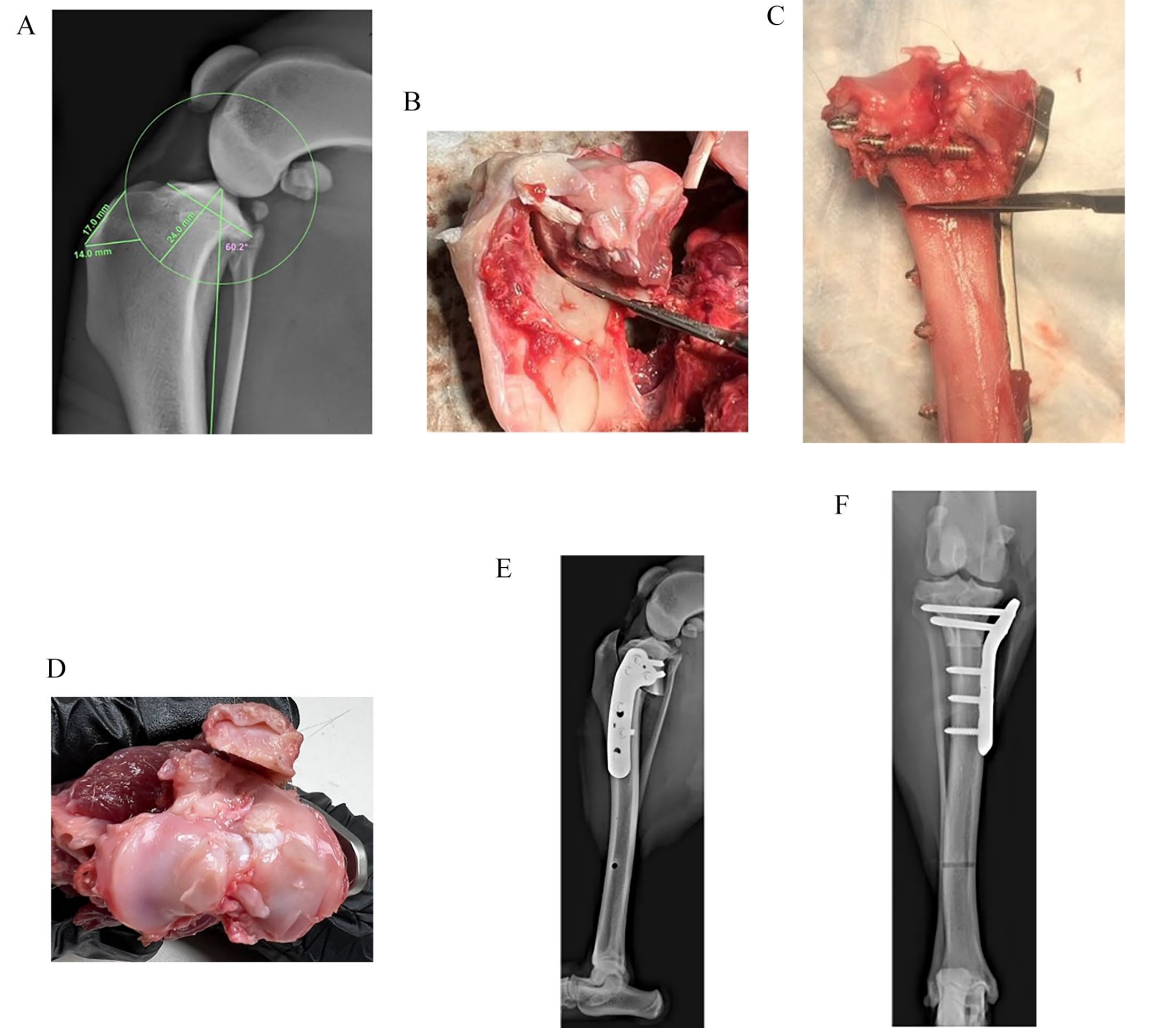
Representative traditional TPLO outcomes and technical errors. (A) Preoperative, calibrated radiographs are taken to determine tibial plateau angle (TPA), saw blade size, desired implant, and D1/D2 measurements. (B) Lateral aspect of proximal tibia revealing an osteotomy gap at the trans cortex. (C) Caudal aspect of proximal tibia revealing an osteotomy gap present at the caudal cortex and a screw present in the caudal popliteal fossa, with minimal bone purchase. (D) Coronal view of tibia demonstrating excessive cranial osteotomy trajectory in the craniocaudal axis. (E) Sagittal and (F) frontal plane postoperative radiographs.
